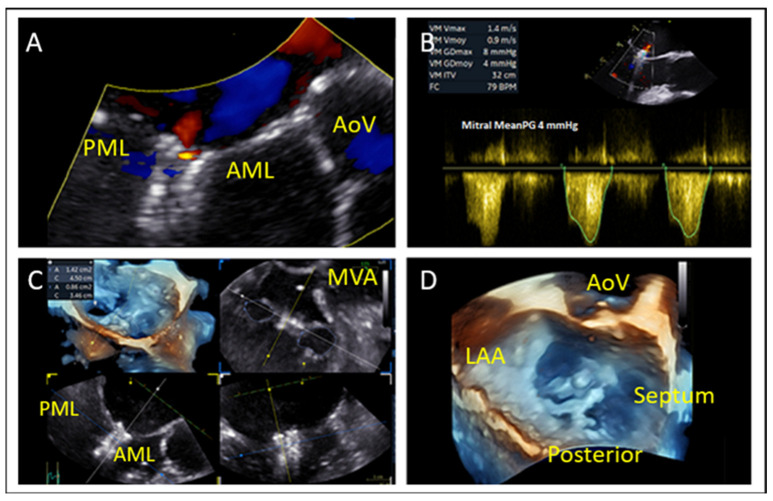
Post-device deployment echocardiographic assessment. (A) Color Doppler imaging reveals trace residual mitral regurgitation, indicating minimal leakage after device deployment. (B) Continuous-wave Doppler evaluation demonstrates a mean trans-mitral gradient of <5 mmHg, indicating the absence of significant iatrogenic mitral stenosis. (C) Three-dimensional multi-planar reconstruction confirms an adequate mitral valve area (MVA ≥ 1.5 cm^2^). (D) Three-dimensional image after device implantation demonstrates good tissue bridging. AoV: aortic valve, LAA: left atrial appendage, AML: anterior mitral leaflet, PML: posterior mitral leaflet, MVA: mitral valve area.
